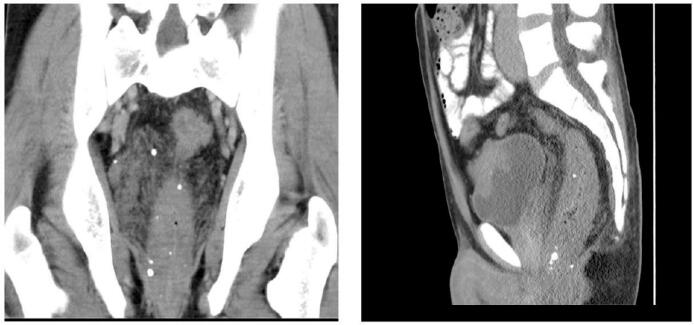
CT scan abdomen and pelvis showed Marked irregular circumferential mural thickening extending from the anorectal wall reaching the distal part of the sigmoid colon, measuring up to 2 cm in thickness and about 15 cm in length, associated with multiple calcifications/phleboliths within the wall and in the mesorectal space along with numerous peri-rectal serpiginous structures.
